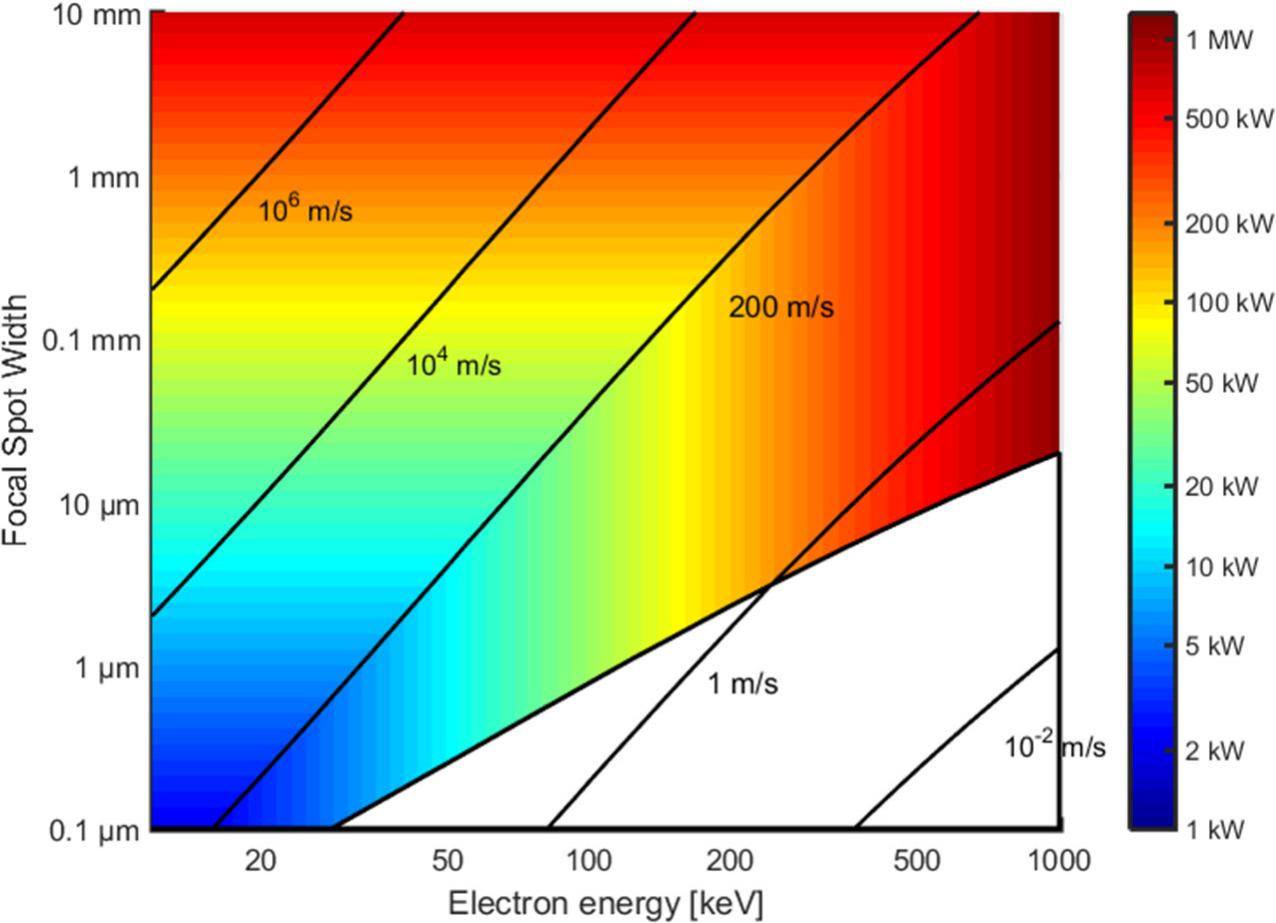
Tube performance at various focal spot widths and acceleration voltages: the maximum possible electron beam power of the x-ray tube is shown in a color scale at a surface velocity of }{}${200}~{\rm m~s^{-1}}$ and a maximum temperature increase of 2500 K in the focal spot (equations (5) and (2)). The isolines show the transition velocity from heat conduction limit to heat capacity limit (equation (6)).
